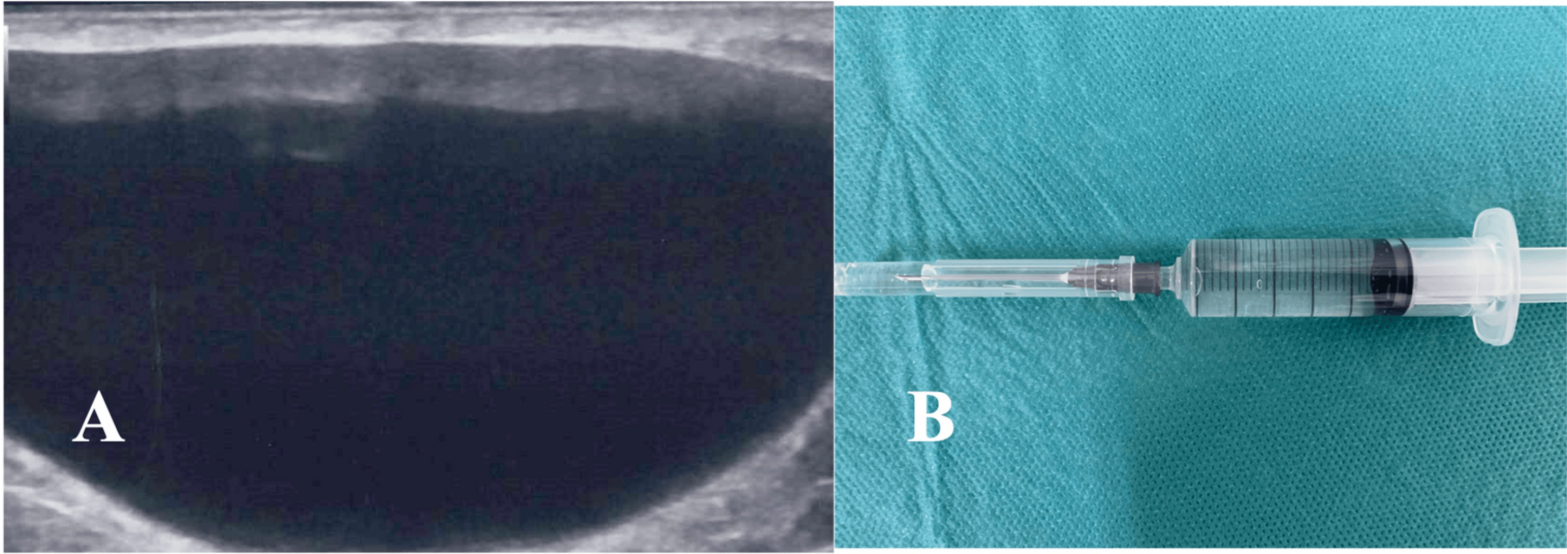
Imaging and Cytology (A) Ultrasonography reveals a cystic lesion beneath the dermis. (B) Fine-needle aspiration yields clear, serous fluid.
